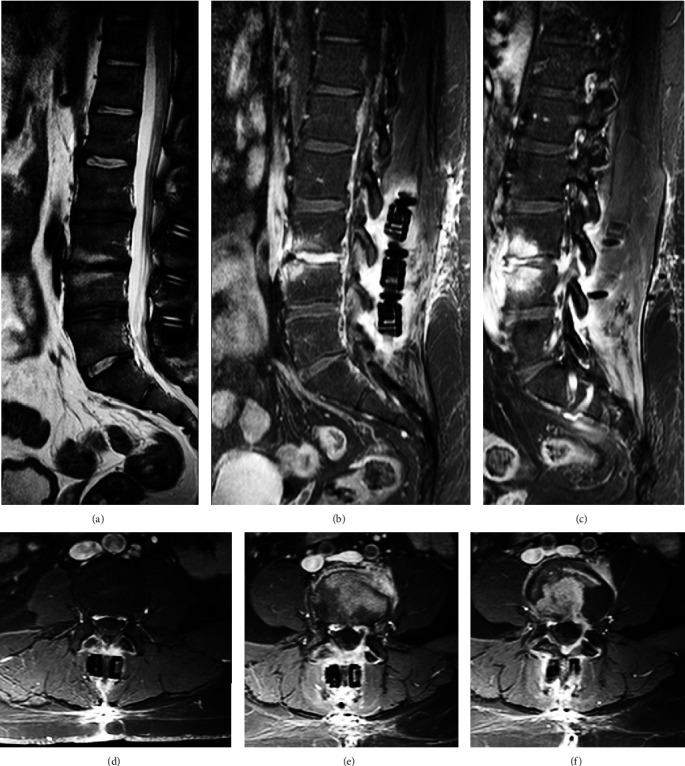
Examples of septic spondylodiscitis on MRI. (a–c) Sagittal view of the lumbar spine. There is a hyperintense signal of the subchondral endplates of L3-L4 on T2 and enhancement after gadolinium administration on T1. There are three consecutive interspinal cages in the interspinal space of L3-L5 surrounded by intense soft tissue enhancement. (d–f) Axial view at the intervertebral level of L3-L5 showing different aspects of discitis and paraspinal tissue enhancement (white arrows).
